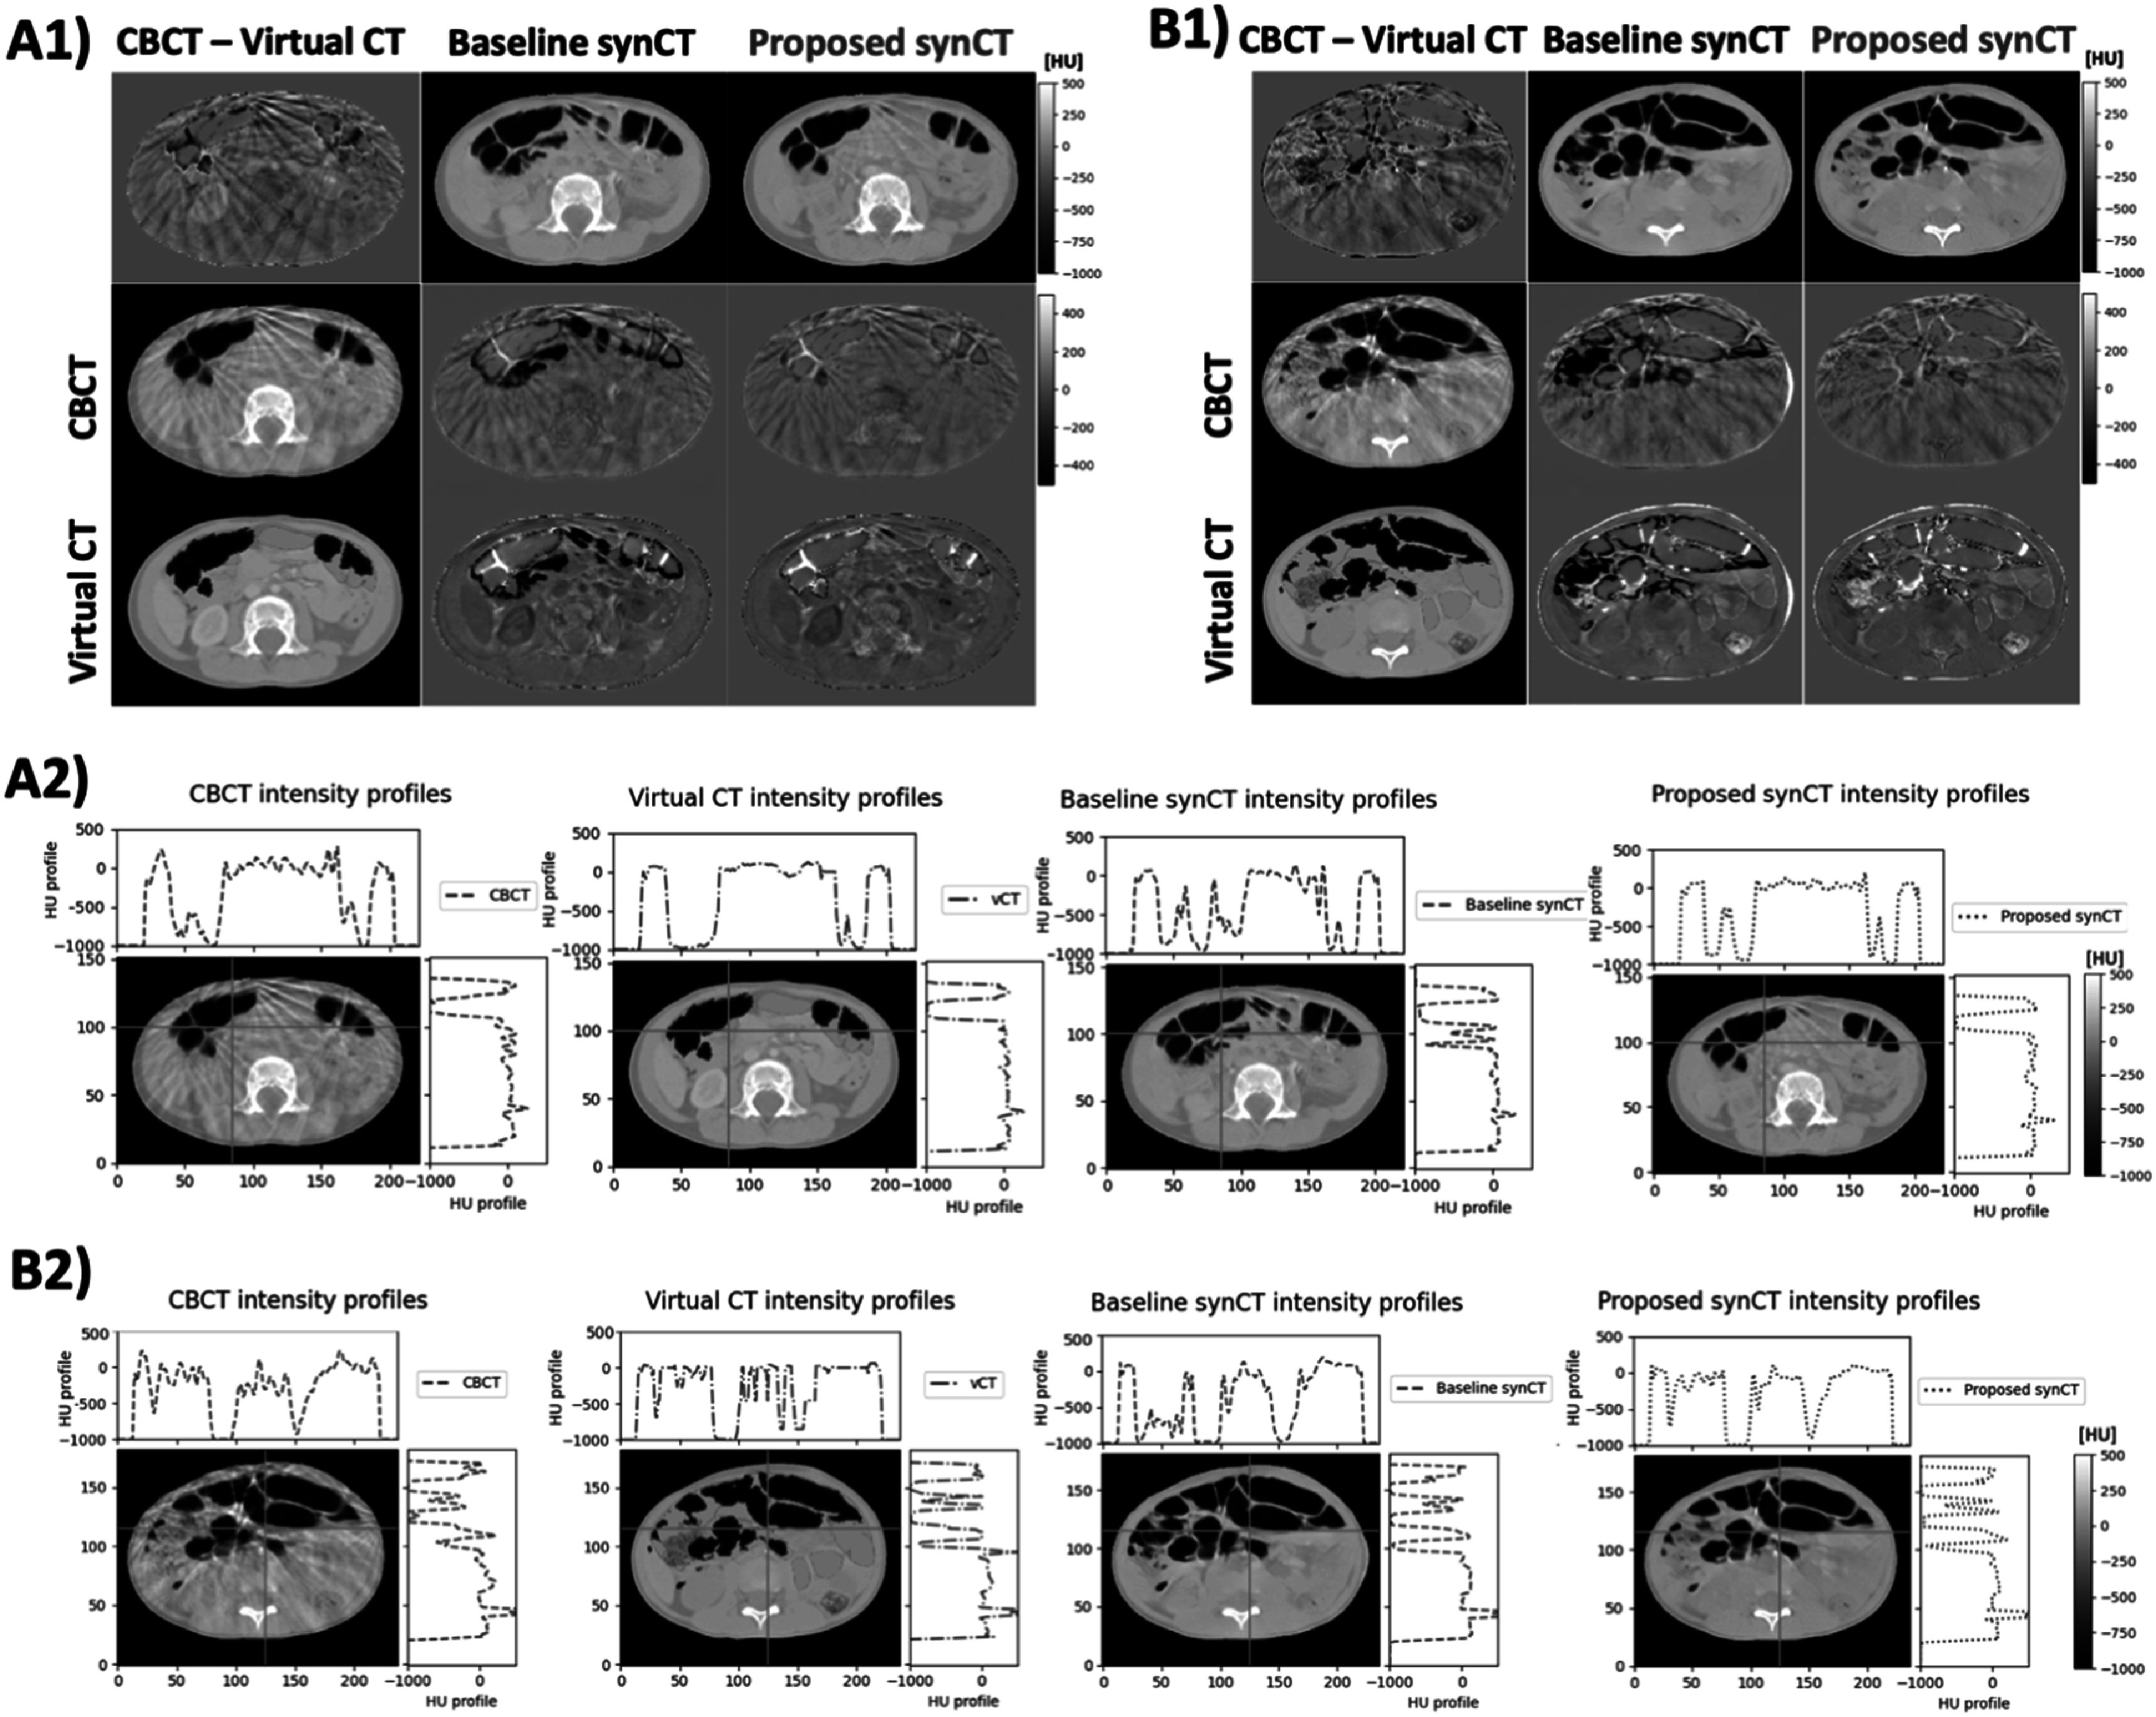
Visual comparison of the results between the proposed and baseline synCTs with respect to the virtual CT and CBCT for two example, cases A and B. The difference images highlight regions of anatomical and intensity disagreement (A1 and B1). The difference between the virtual CT and CBCT highlights the challenge in establishing reliable ground-truth images. For both cases, intensity profiles for cross-sections for the CBCT, virtual CT, baseline synCT and the proposed synCT are also shown (A2 and B2).
